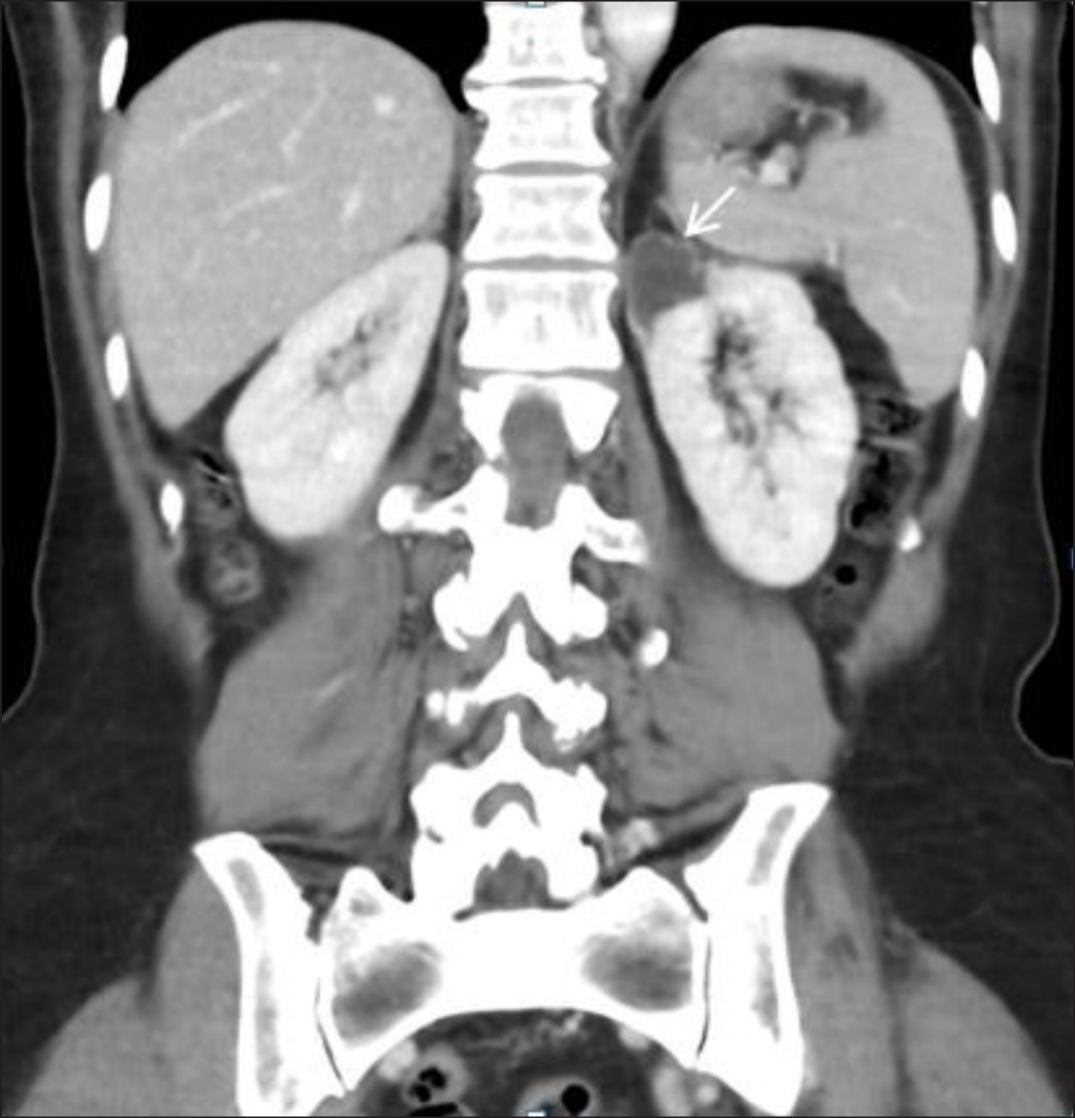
Coronal view of a contrast-enhanced computed tomography shows the upper renal moiety, which is dilated. Due to long-standing hydronephrosis in the upper pole there is a significant secondary parenchymal loss (white arrow).
